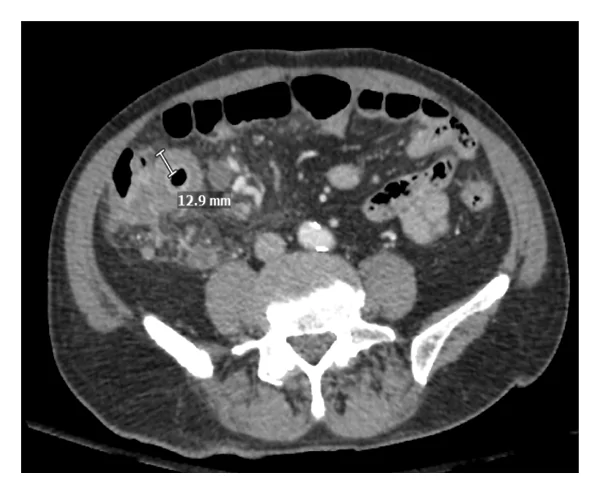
Thickening of the terminal ileum measuring 12.9 mm seen on CT of the abdomen and pelvis with contrast consistent with terminal ileitis.
